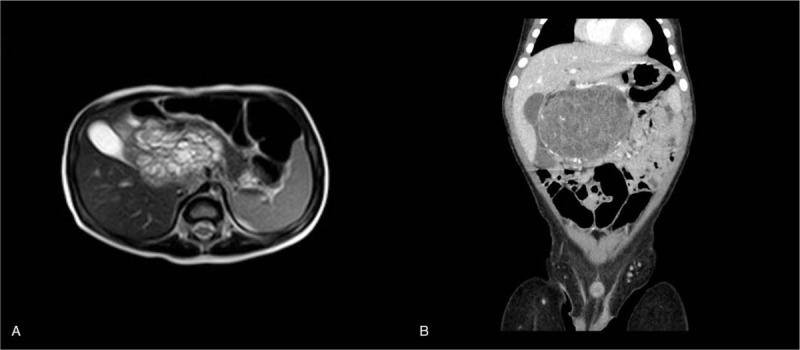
(A, B) Preoperative contrast-enhanced CT scan of the pediatric pancreatic teratoma (A B). The cystic solid mass in the right retroperitoneal region of the mid-upper abdomen is mainly cystic, with honeycomb septation and patchy shadow. The mass is closely related to the head of the pancreas. CT = computed tomography.
